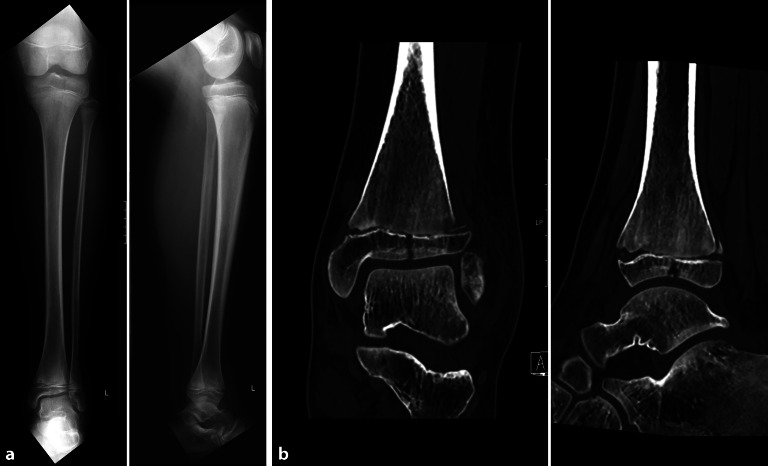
In der CT können zudem mit KontrastmittelgabeKontrastmittelgabe angiographische Gefäßrekonstruktionen dargestellt werden. Moderne Dual-Energy- und Photon-Counting-CT ermöglichen spezifische GewebecharakterisierungenGewebecharakterisierungen wie Gichtdarstellungen, virtuelle Nativsequenzen, aber auch Knochenödemdarstellungen. Die StrahlenbelastungStrahlenbelastung in der CT hat mit den aktuellen CT-Generationen durch Einsatz von iterativen Rekonstruktionsmöglichkeiten, automatischer Dosismodulation, modernen Detektoren, Filtern und neuerdings Photon-Counting-Detektoren signifikant abgenommen bei besserer Bildqualität.
